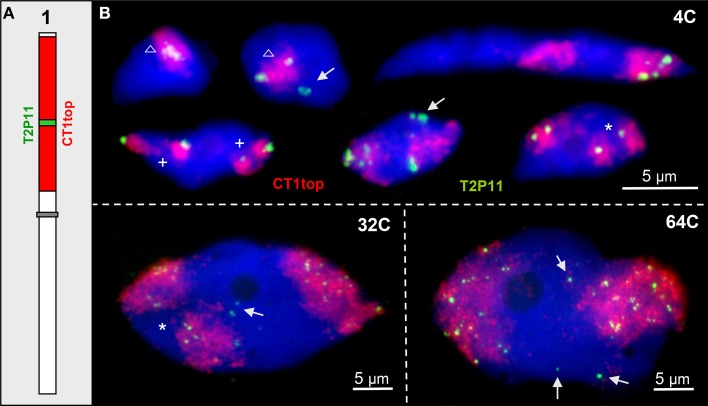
~85 kb euchromatin segments may loop out from their CTs. (A) Scheme of chromosome 1 showing the labeling of CT1top arm, and BAC T2P11 therein in different color as used for FISH. (B) Examples of chromatin configurations in 4C, 32C, and 64C nuclei. Sister chromatid territories of one (asterisks) or both (cross) homologs may be separated due to missing chromosome arm cohesion. The T2P11 segment is associated with (triangle) or separated from its CT (arrows). The strongly elongated 4C nucleus shows the T2P11 segment absent from the left CT and moved into the homologous right CT.
